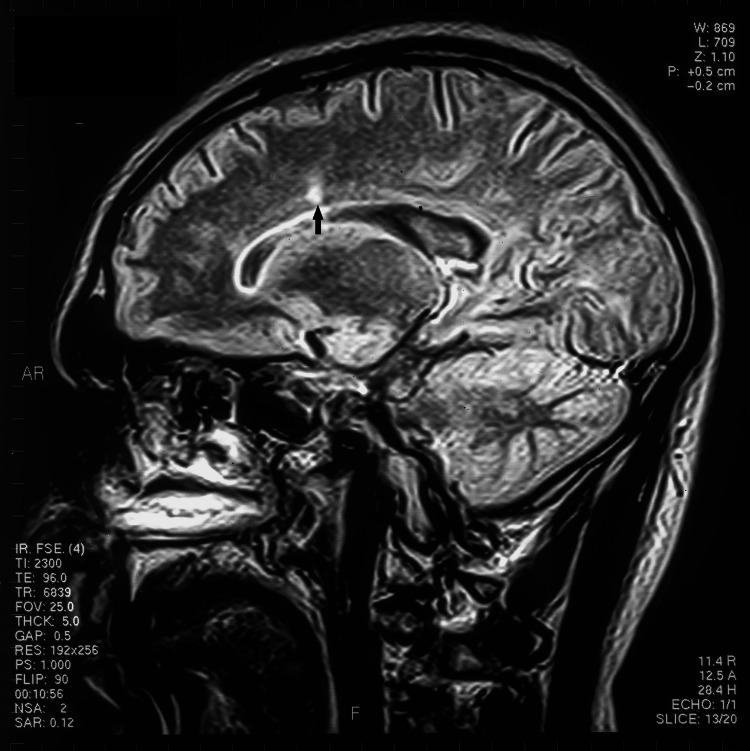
T1 sagittal MRI arrow showing an area of demyelination adjacent to the corpus callosum
